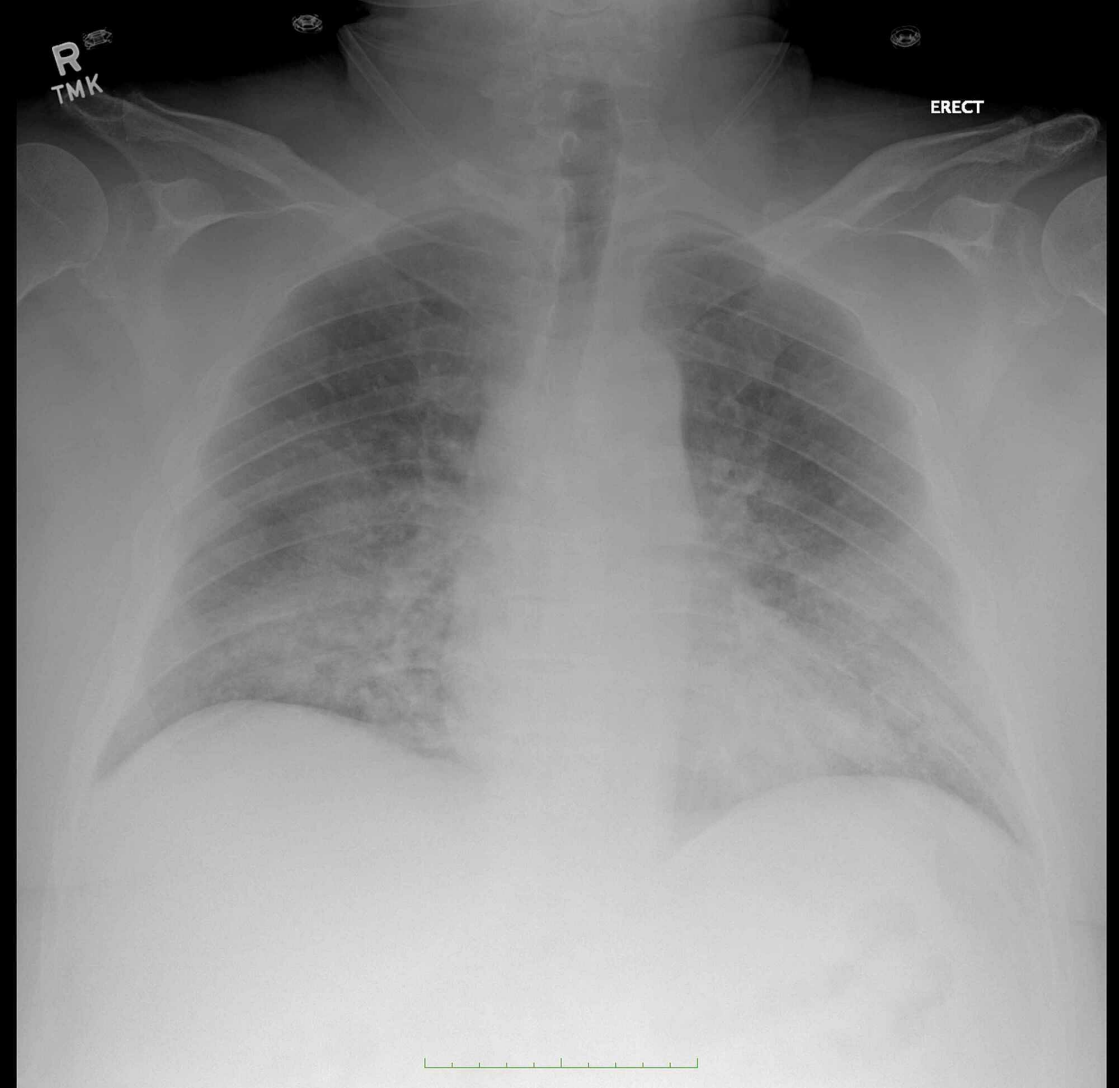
Chest X-ray during the first emergency visit showing bilateral pulmonary infiltrates and bilateral lung airspace disease suggesting edema or pneumonia
